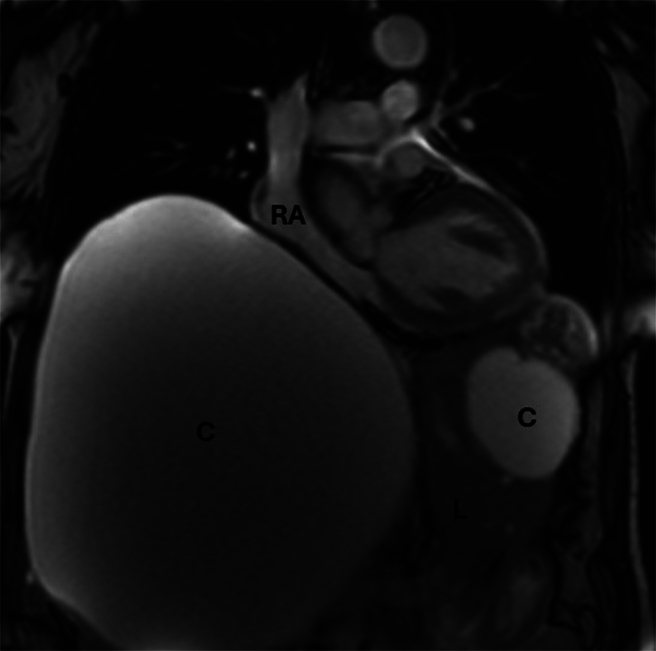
Abdominal MRI revealed two hepatic cysts (C), the massive one (190×210mm) compressing the right atrium (RA) and liver (L). Both cysts appeared as simple hepatic cysts radiologically. MRI = magnetic resonance imaging.
